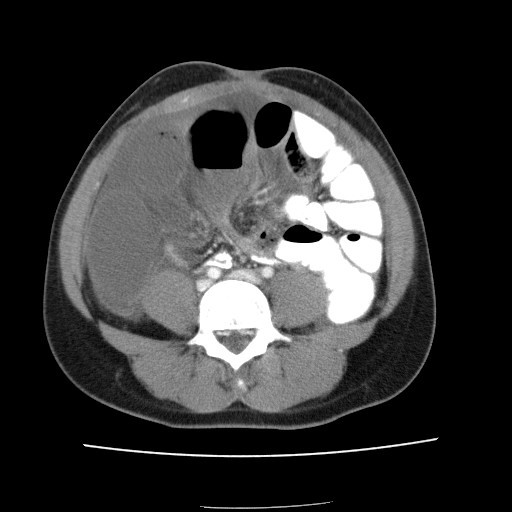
CT showing dilated proximal jejunum and a closed loop obstruction in the terminal ileum. There is a positive "swirl" sign consistent with a diagnosis of small bowel volvulus.
